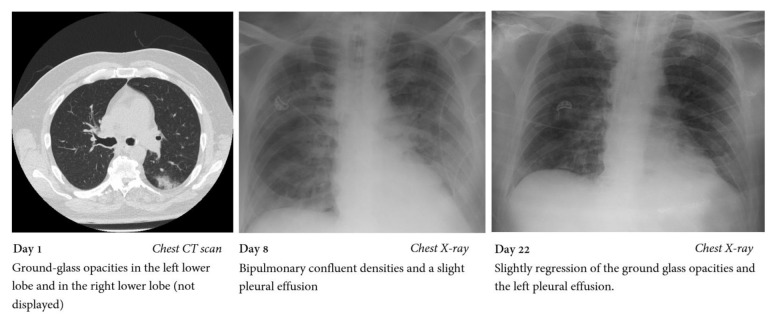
Chest CT scan and chest X-rays performed during hospital stay.
